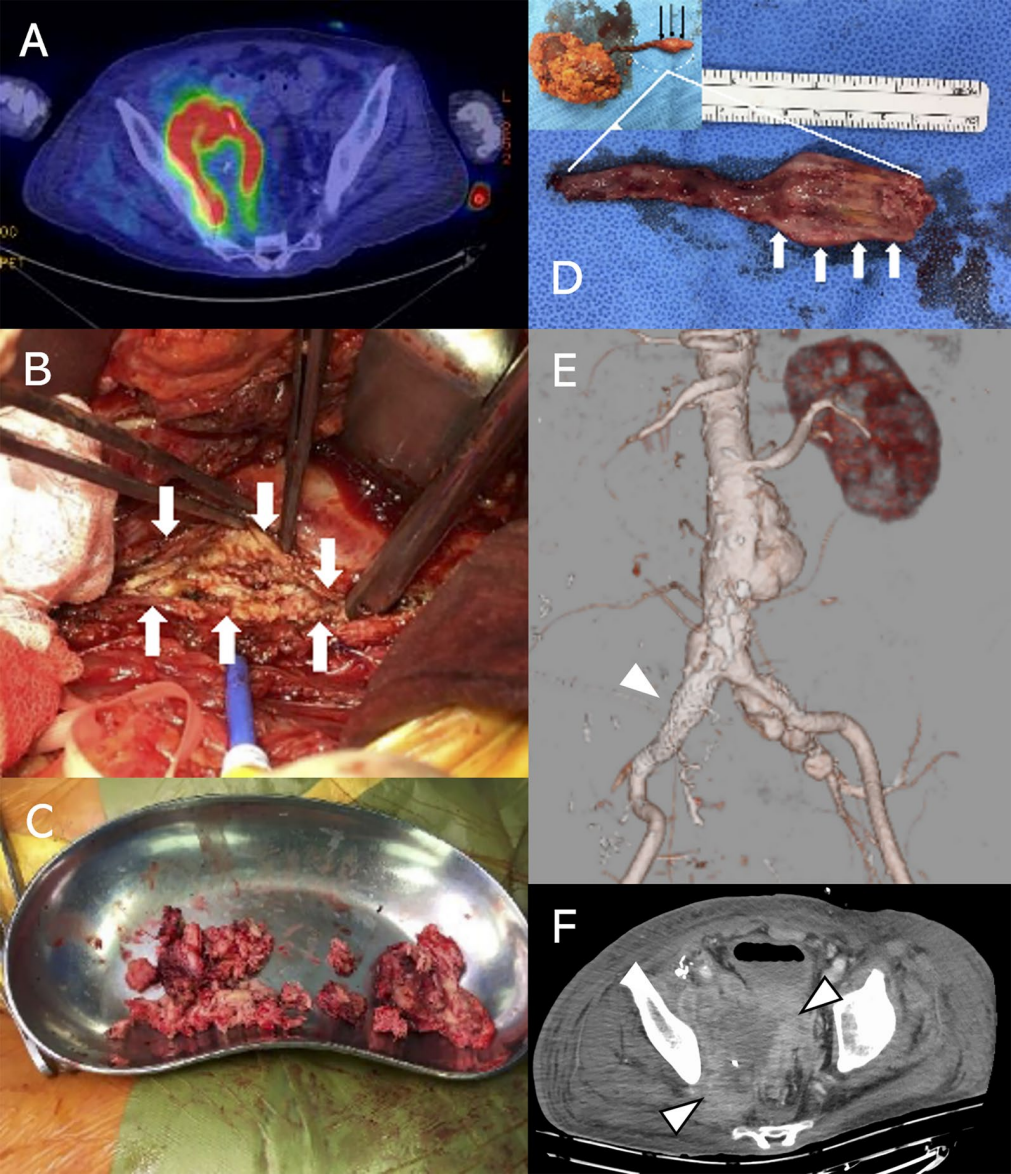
A Positron emission tomography–computed tomography (CT) revealed accumulation consistent with retroperitoneal fluid retention around the aneurysm. B Intraoperative image of the first laparotomy. The iliopsoas muscle (arrows) had a white cottage cheese-like secretion inside. C, D Intraoperative image of the second laparotomy. The white necrotic tissues were resected to control infection. The right kidney and ureter were also resected. The distal part of the ureter was enlarged (thin black arrows). No purulent discharge was observed in the lumen, and only wall thickening (thick white arrows) was noted. E Postoperative CT angiography after the second laparotomy revealed complete isolation of blood flow in the IIA by endovascular aortic aneurysm repair (EVAR, arrowhead). F Pelvic mass grew despite EVAR (arrowheads)
